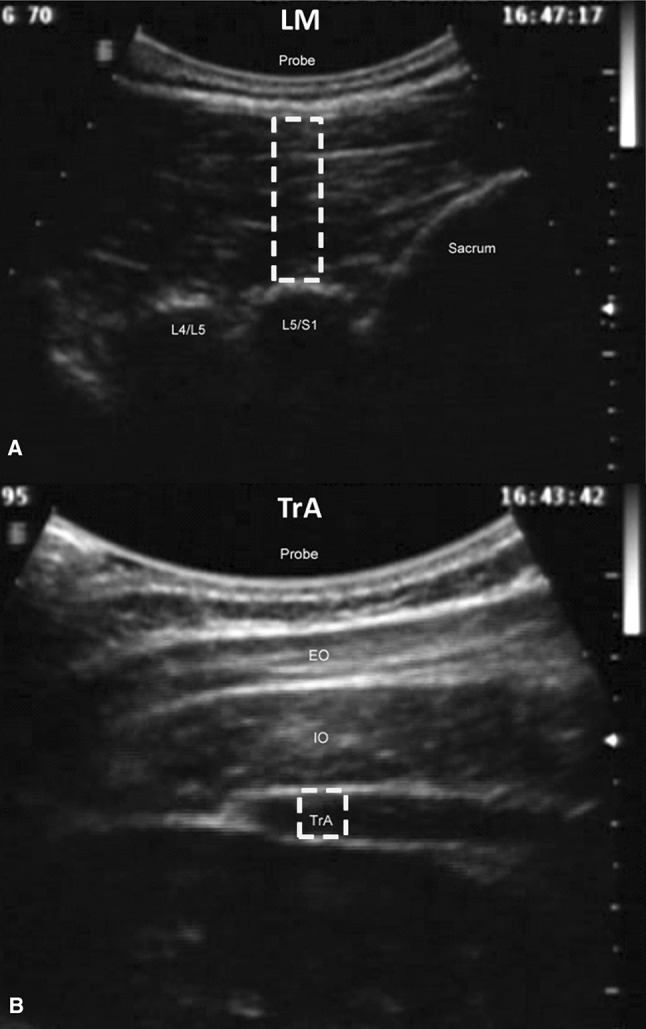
Typical LM (a) and TrA (b) ultrasound image; the probe, sacrum, L5/S1 and L4/L5 facet joints are labelled. The *white rectangle* shows the location where the area of interest was positioned for automatic edge detection
